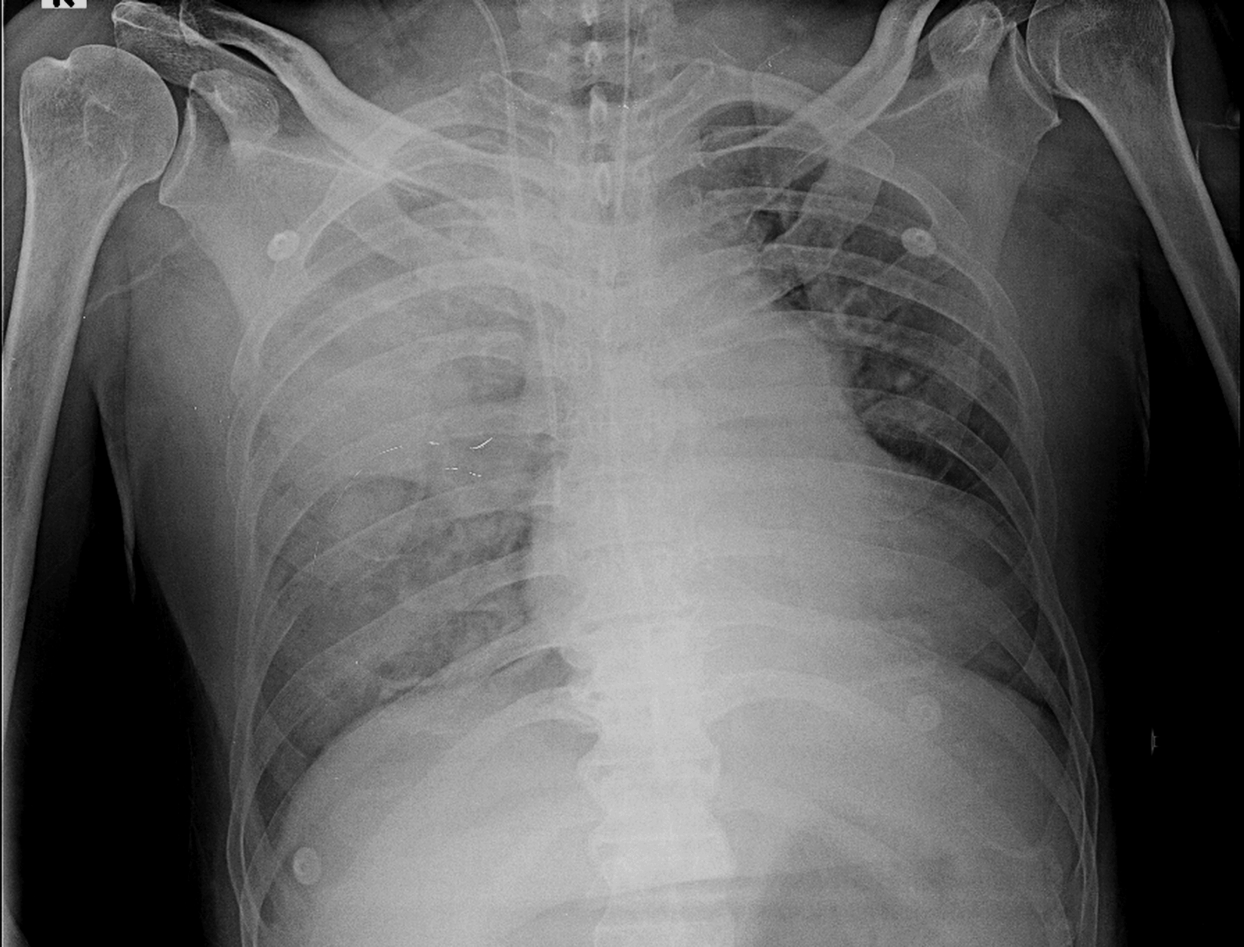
Portable anteroposterior chest radiograph at the emergency department presentation A portable anteroposterior (AP) chest radiograph shows extensive right-sided air-space consolidation. No definite visceral pleural line is identified and there is no radiographic evidence of pneumothorax on this film (noting the reduced sensitivity of portable AP projection). An endotracheal tube is in situ; a central venous catheter is present with the tip projecting over the lower superior vena cava/cavo-atrial junction. External monitoring electrodes are visible.
